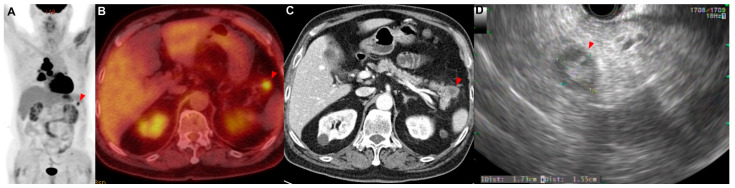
Patient 1: a 71-year-old man diagnosed with non-small-cell lung cancer (NSCLC) with a solitary metastasis in the tail of the pancreas. (A–C) Positron emission tomography/computed tomography (PET/CT) showed a solitary metastatic lesion (red arrowhead) in the tail of the pancreas. (D) EUS-FNA was performed at the metastatic lesion (red arrowhead) in the pancreas.
